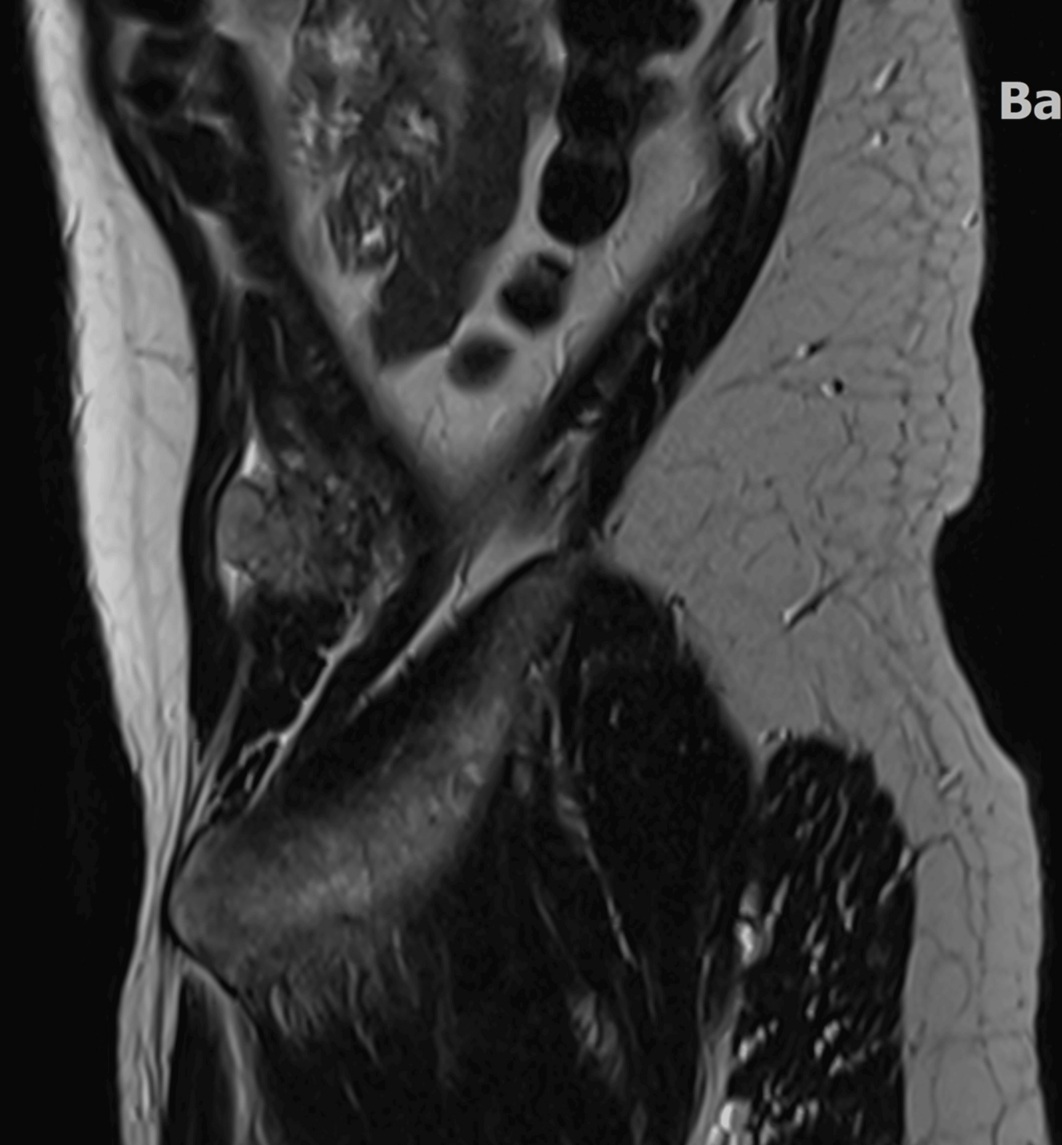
MRI sagittal T2 without FAT SAT (fat-saturation) Heterogeneous hyperintensity T2 space occupying the mass in the left internal oblique muscle.
